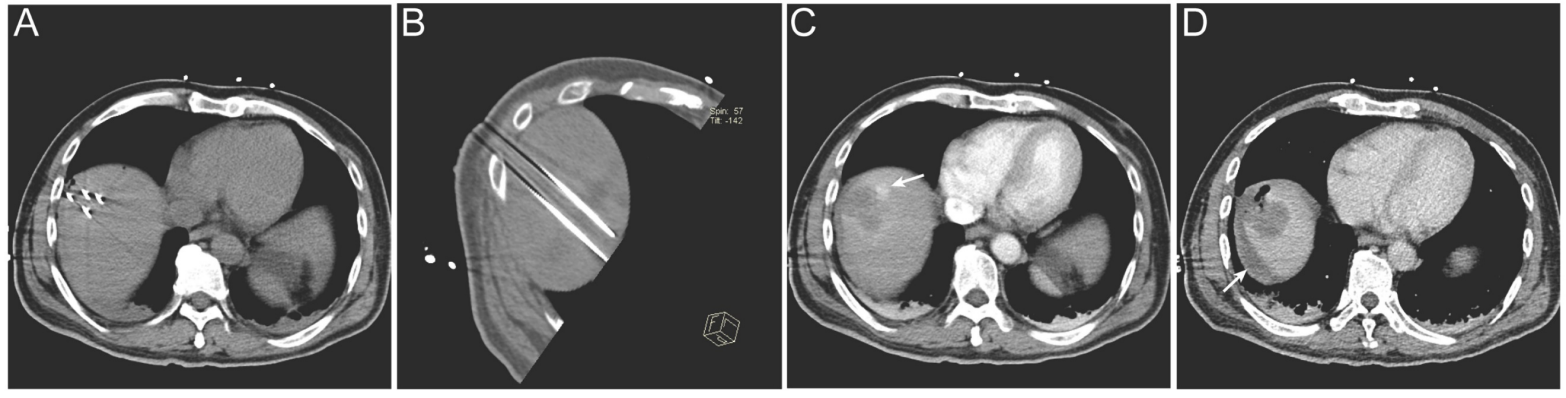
Representative imaging of procedure-related complication following IRE treatment. (A, B) Intra-procedural electrode placement shown on an axial CT image (A) and an oblique multiplanar reconstructed (MPR) CT image (B); (C) Immediate post-ablation arterial-phase contrast-enhanced CT demonstrating focal contrast extravasation at the ablation margin (arrow); (D) Post-ablation delayed-phase contrast-enhanced CT demonstrating perilesional fluid attenuation (arrow).
